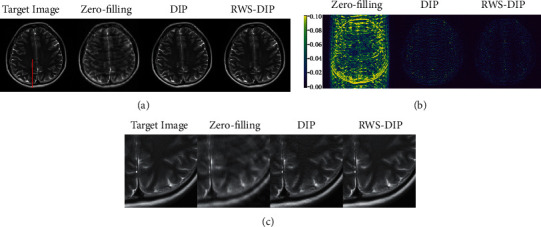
Comparison of reconstructions of the target MR image in Brain C using Cartesian undersampled mask with 30% sampling rate: (a) the target image and reconstruction results, (b) the corresponding error images, and (c) the corresponding zoom-in images.
